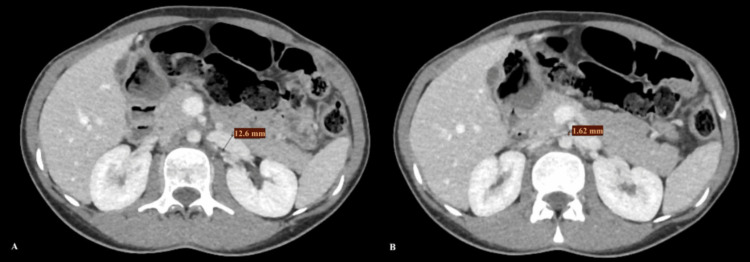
Axial plane intravenous contrast-enhanced computed tomography, demonstrating the compression of the left renal vein by the SMA, with a compression ratio indicating nutcracker syndrome (A) Prestenotic dilation measuring 12.6 mm. (B) Compressed segment measuring 1.62 mm SMA: superior mesenteric artery
